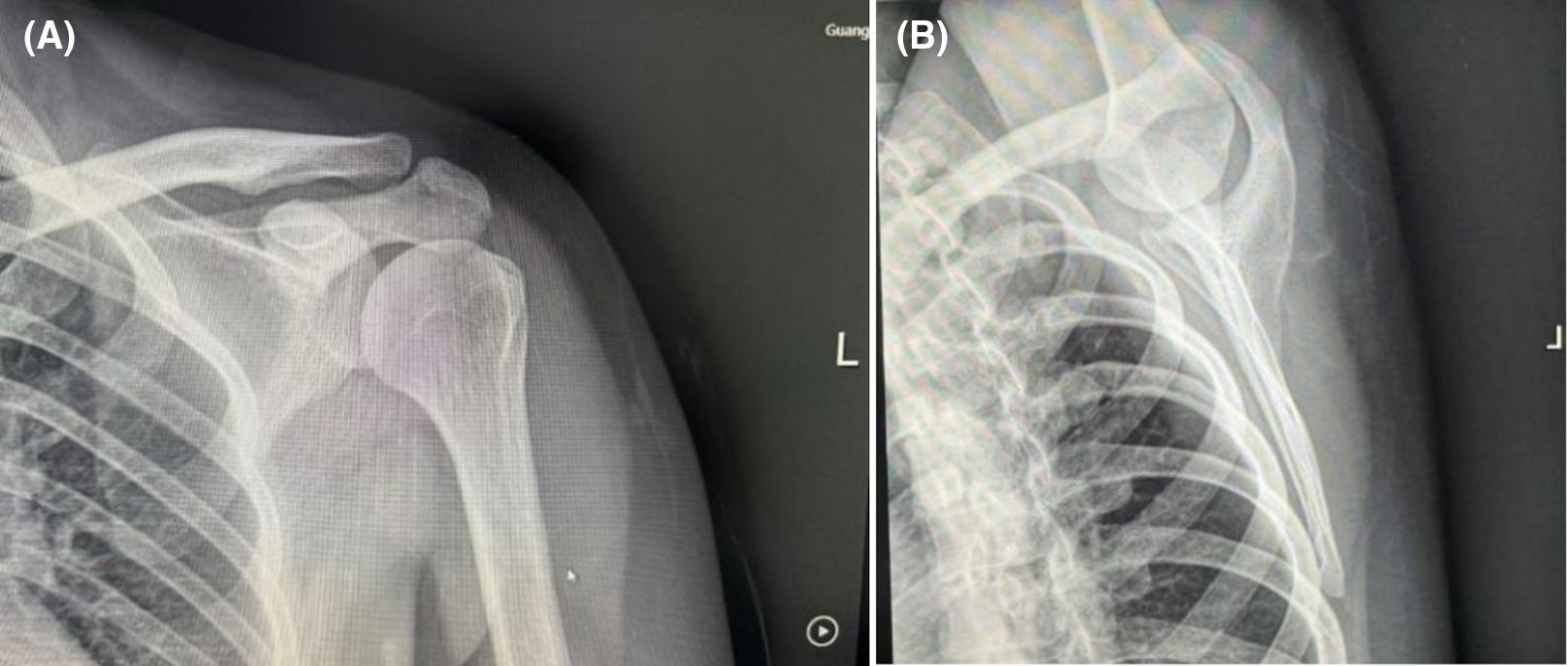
(A) & (B) Radiographs one‐year after surgery showed that the osteochondroma was completely resected.
